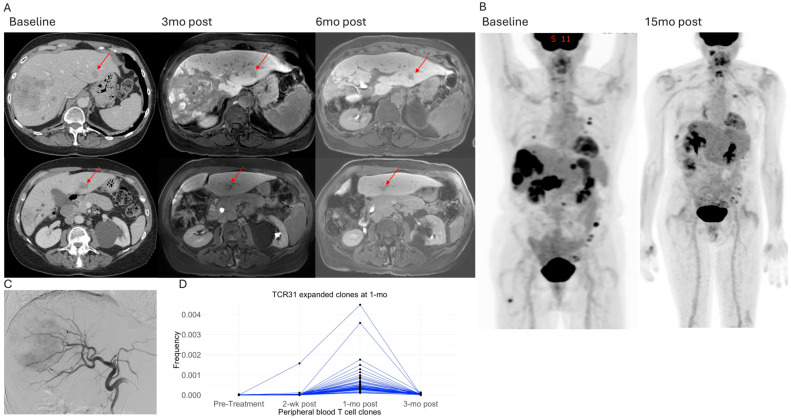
T-cell clonal expansion for a patient with metastatic uveal melanoma treated by Y90 (TCR31). (A) Baseline CT and follow-up MRI 3-month and 6-month after Y90. The red arrows annotate left lobe tumors (a segment 2 tumor in upper row, and a segment 3 tumor in lower row) not treated by Y90 which decreased size over time. (B) PET/CT imaging at baseline and 15 months after Y90 treatment demonstrated significantly decreased FDG uptake in the treated tumor within the right lobe. Additionally, the previously FDG-avid tumors in the left lobe and multiple extrahepatic sites at baseline showed no FDG avidity at the 15-month follow-up. (C) Angiogram at time of Y90 to the right lobe showed the dominant tumor. (D) Frequency tracking of T-cell clonotypes expanded 1 month after Y90-RE. Each line represents the clonal frequency of a single T-cell clonotype.
